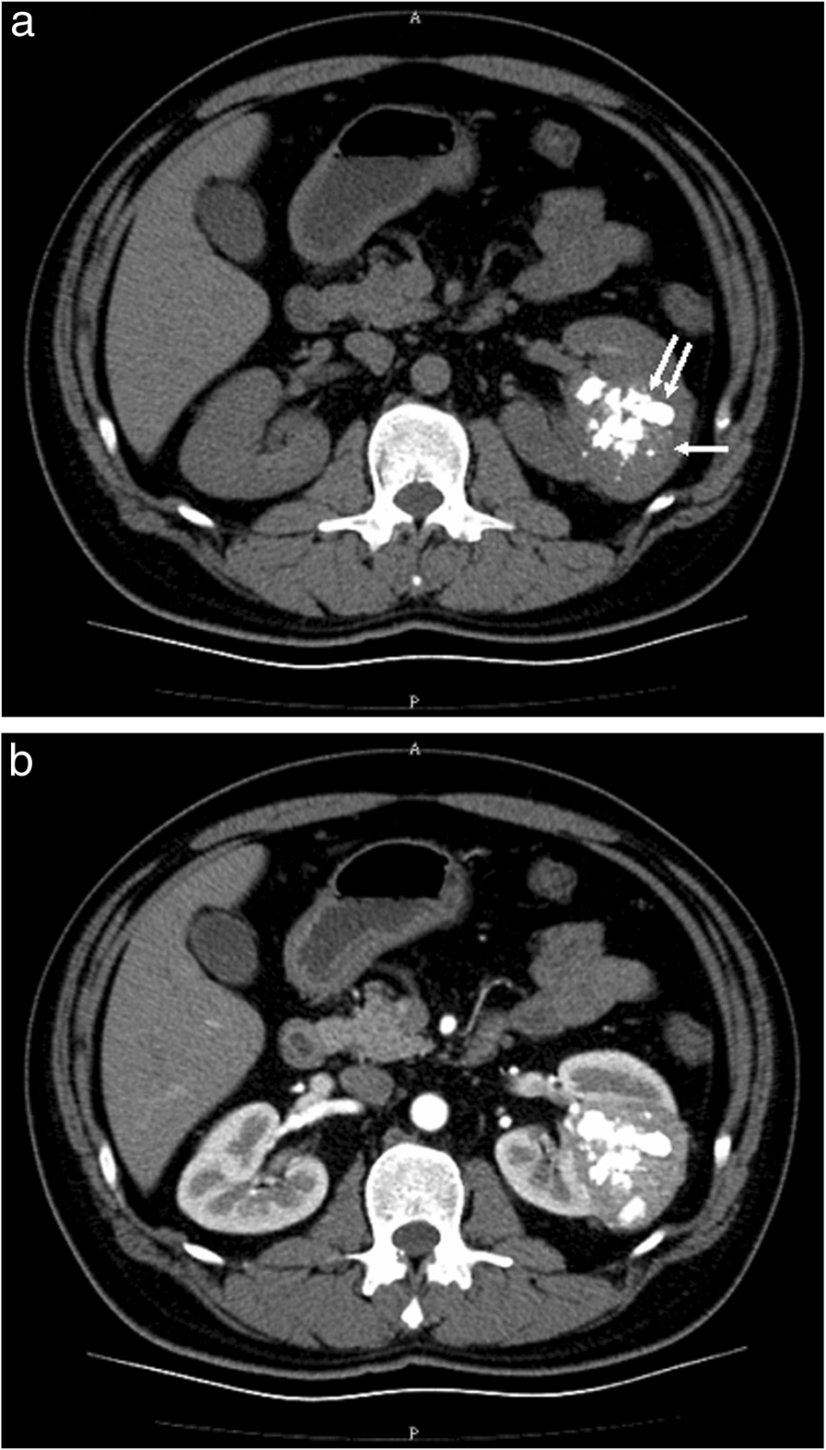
CT scan of the angiomyolipoma (AML) of the left kidney. (a) Noncontrast scan showing a solid tumor (arrow) with multiple calcifications (double arrow). (b) Contrast CT imaging showed contrast enhancement.
